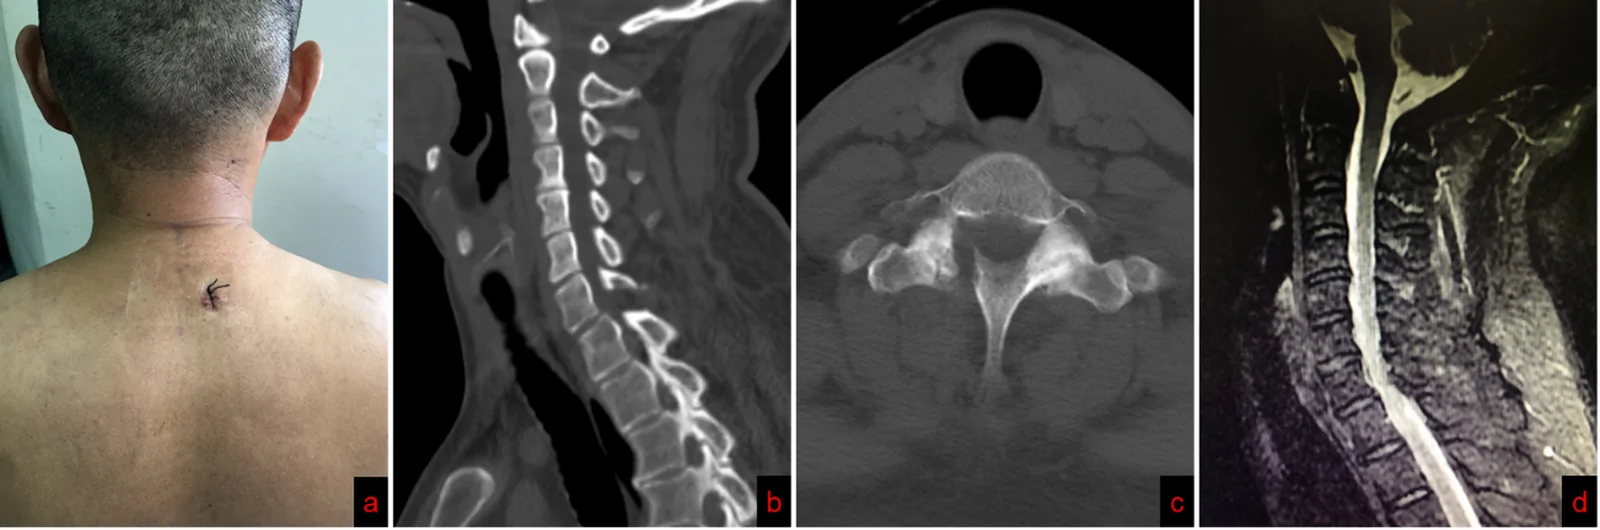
a Postoperative incision status. b, c Postoperative CT scan images. d Postoperative MRI scan images
